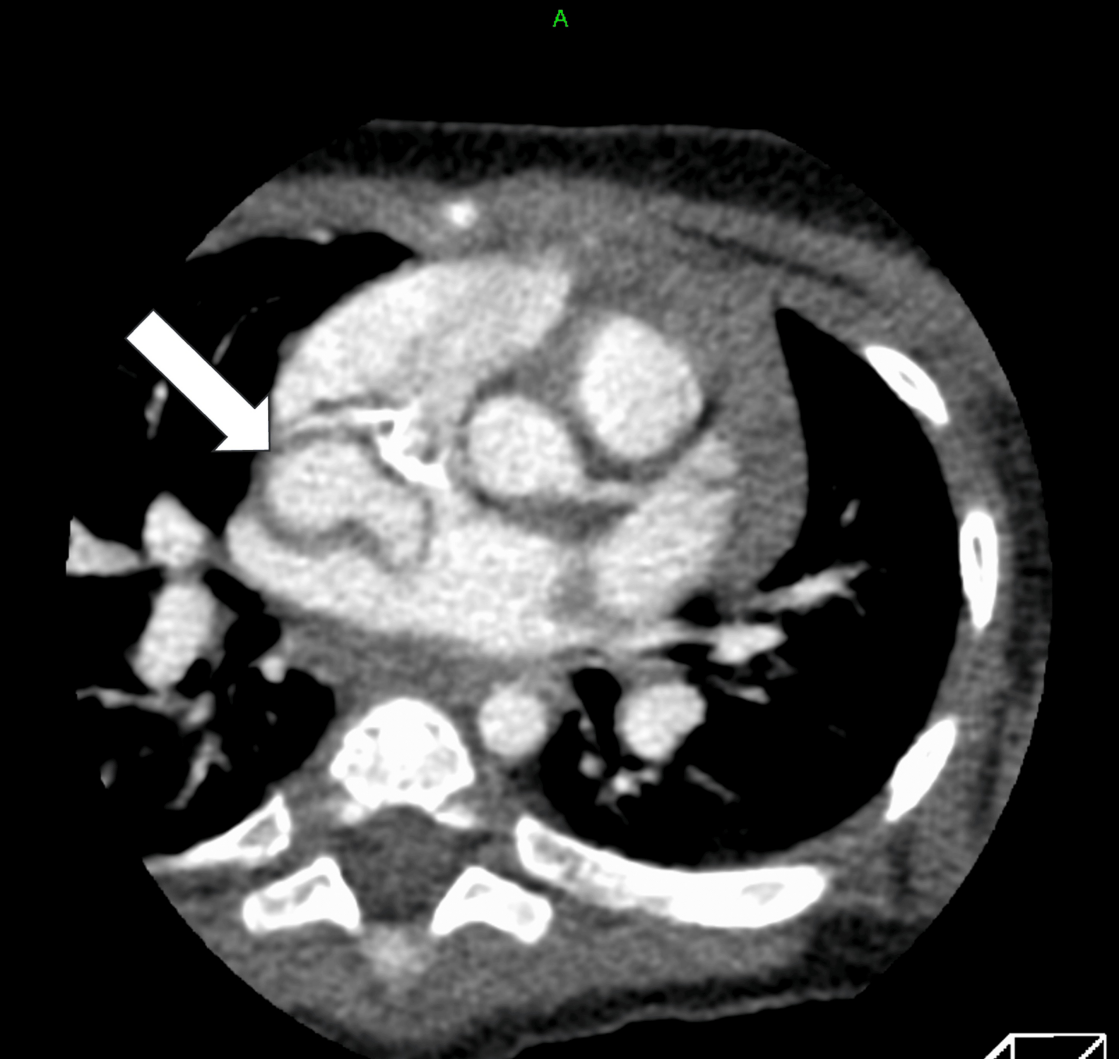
Contrast-enhanced computed tomography (axial image) Contrast-enhanced computed tomography (axial image) demonstrated an aneurysm between the right atrium and the right pulmonary vein.
